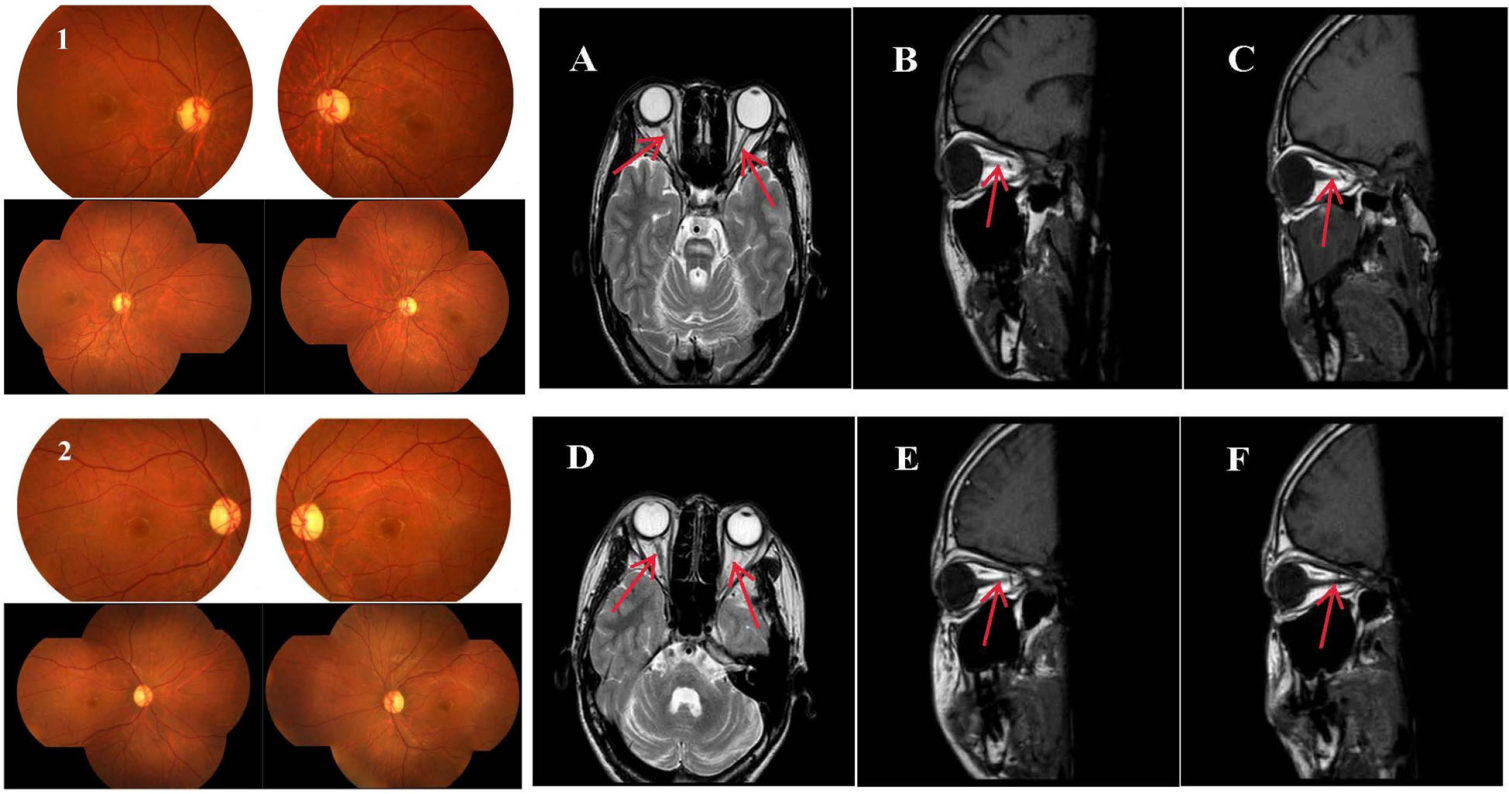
Fundus photography and periorbital magnetic resonance images of two patients. (1) Shows the fundoscopic results of patient 1, and (2) shows the fundoscopic results of patient 2. Both figures show optic disc diffused pallid bilaterally without diabetic retinopathy. The two patients’ optic nerves were pale, and the bilateral optic nerves were thinner. A-C Bilateral optic nerve atrophy from the coronal and sagittal positions in patient 1. D-F Bilateral optic nerve atrophy from the coronal and sagittal positions in patient 2. Red arrows show the atrophic optic nerves of the two patients
